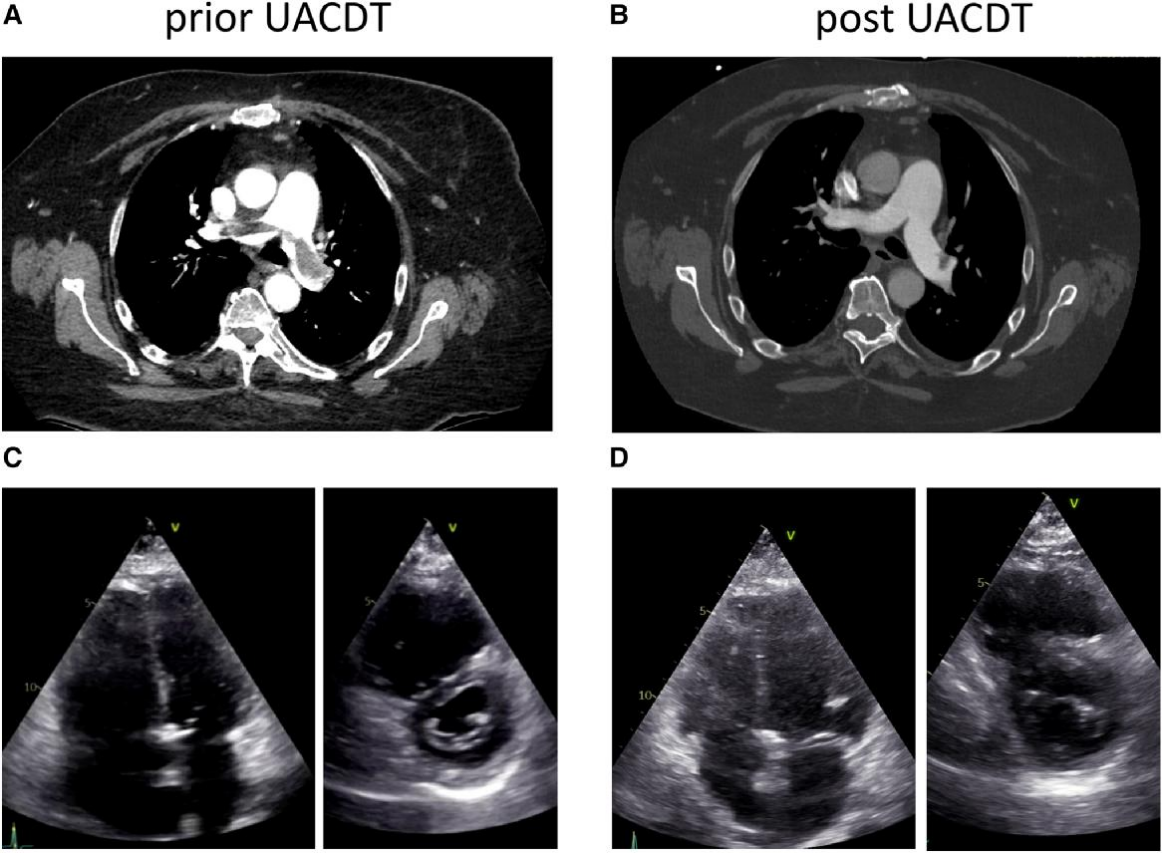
Computed tomography pulmonary angiography and transthoracic echocardiography prior and post ultrasound-assisted catheter-directed thrombolysis. Computed tomography pulmonary angiography prior (*A*) and post (*B*) ultrasound-assisted catheter-directed thrombolysis indicating nearly complete resolution of pulmonary thrombus. Transthoracic echocardiography prior (*C*) and post (*D*) ultrasound-assisted catheter-directed thrombolysis indicating resolving signs of right heart dysfunction. UACDT, ultrasound-assisted catheter-directed thrombolysis.
